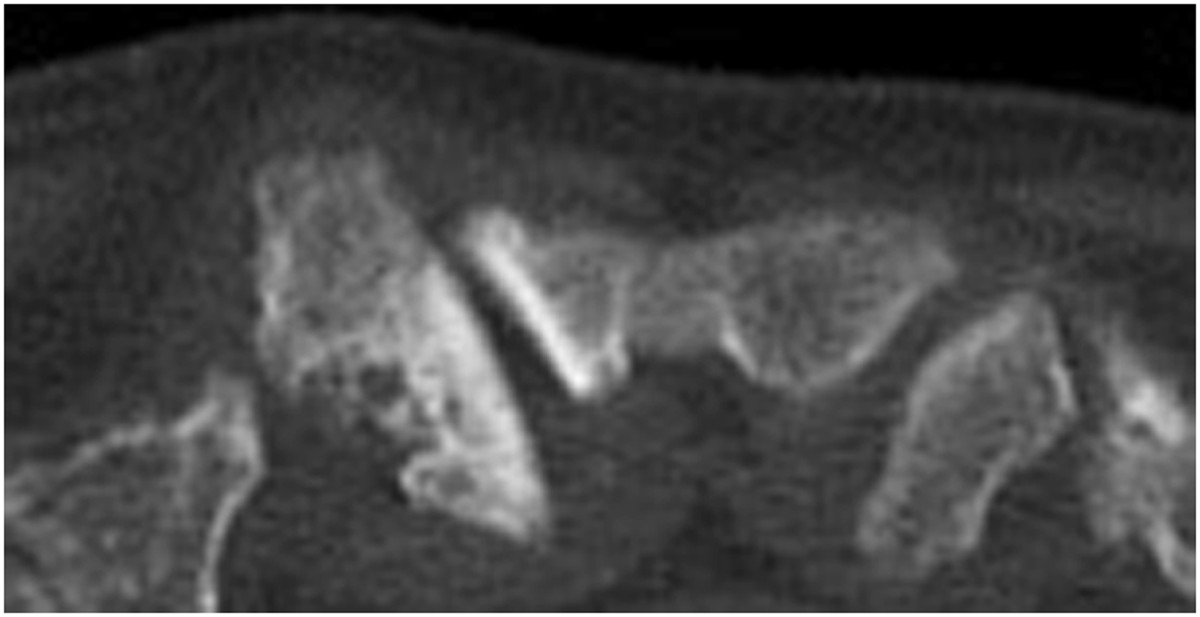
Axial CT image in patients with symptomatic degenerative SC arthritis.
